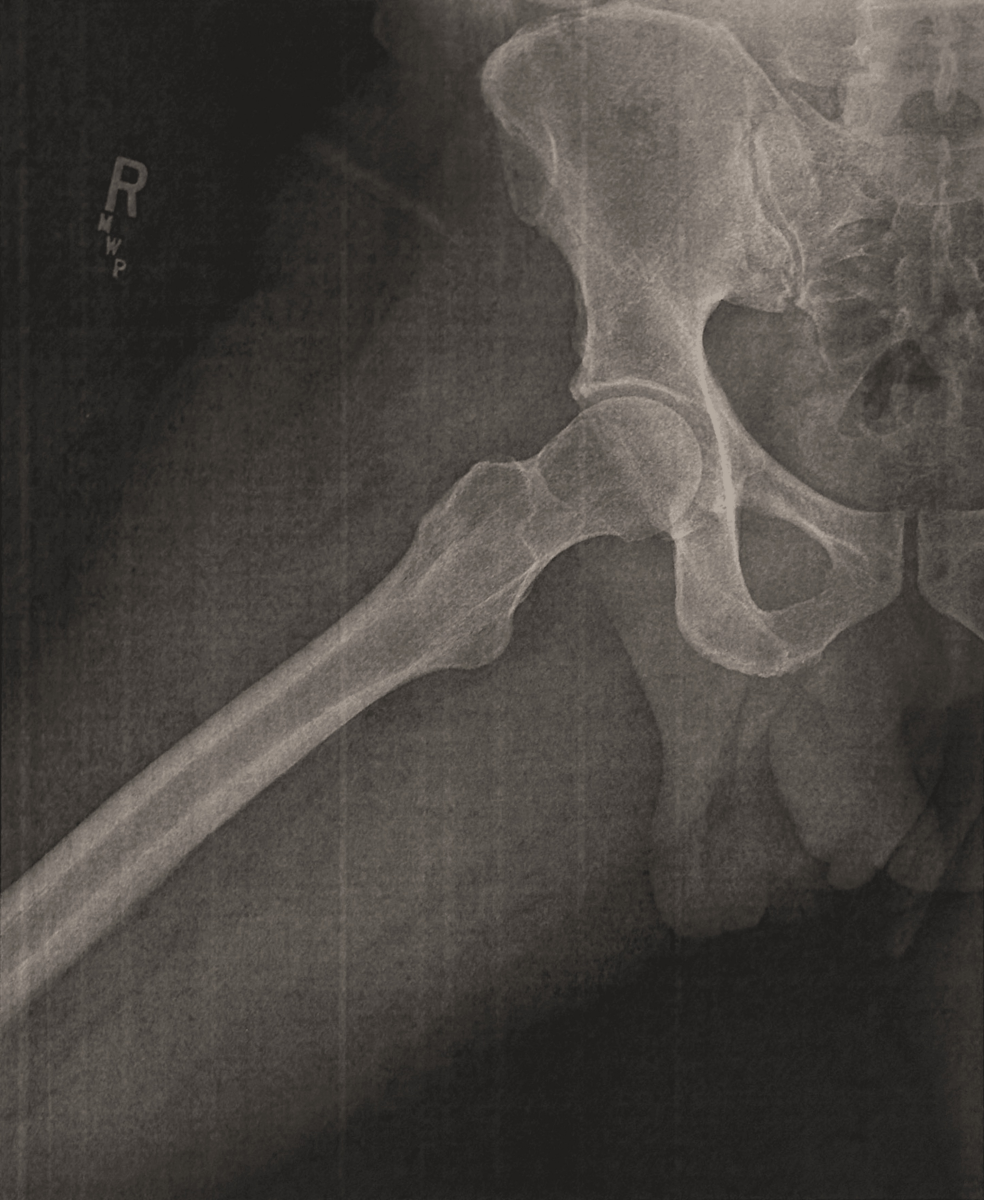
X-Ray of the Right Hip (Month 1) No significant clinical findings.
